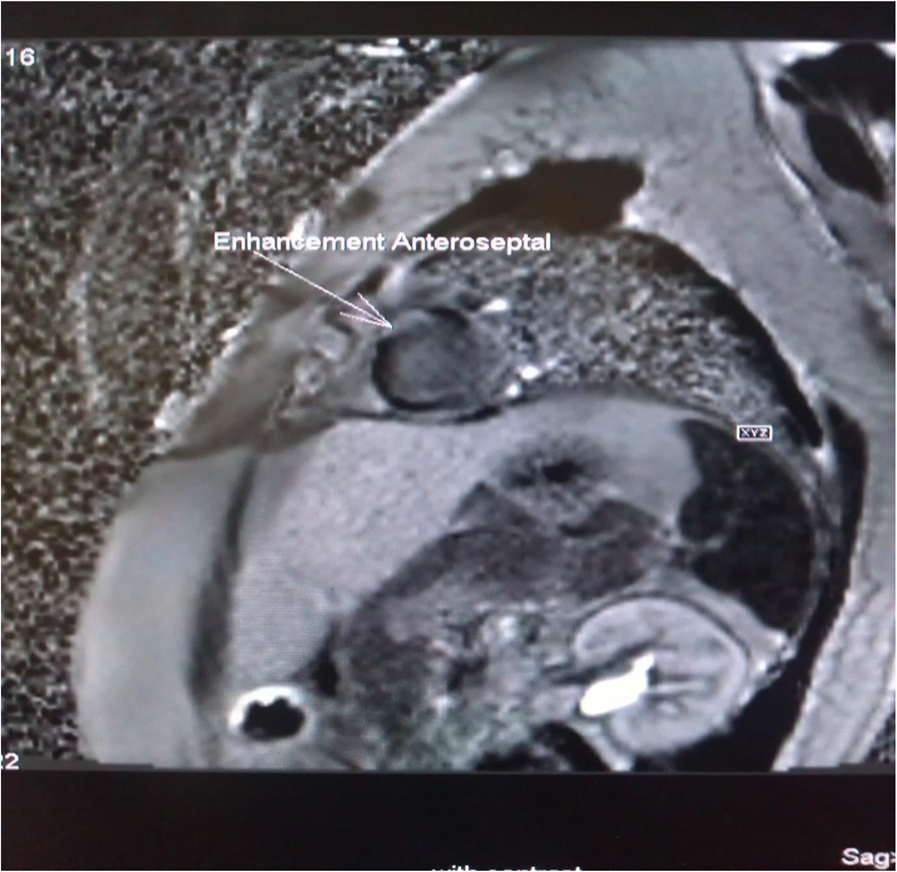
Cardiac magnetic resonance imaging (contrast enhanced) showing enhancement of anteroseptal left ventricular region (long arrow).
